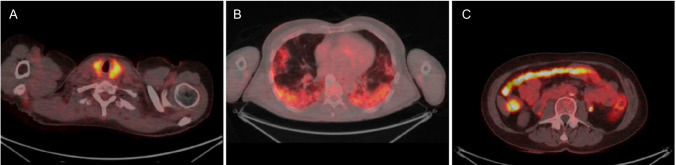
Adapted and modified from Gideonse et al. 2024 [30]. The FDG-PET/CT images of three patients who were diagnosed with immune-related adverse events (irAE) following immunotherapy. A Thyroiditis, B pneumonitis, and C colitis
